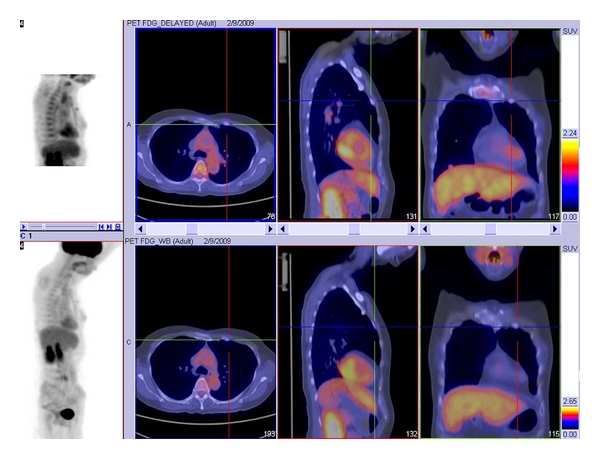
18F-FDG-PET/CT of a 57-year-old woman with a 10-year history of widely invasive follicular thyroid carcinoma. Serum thyroglobulin was elevated to 121 ug/L (normal < 55 ug/L), but both diagnostic whole-body ^131^I scan and ultrasound were negative. The FDG-PET/CT revealed hypermetabolic FDG uptakes in the rib cage.
